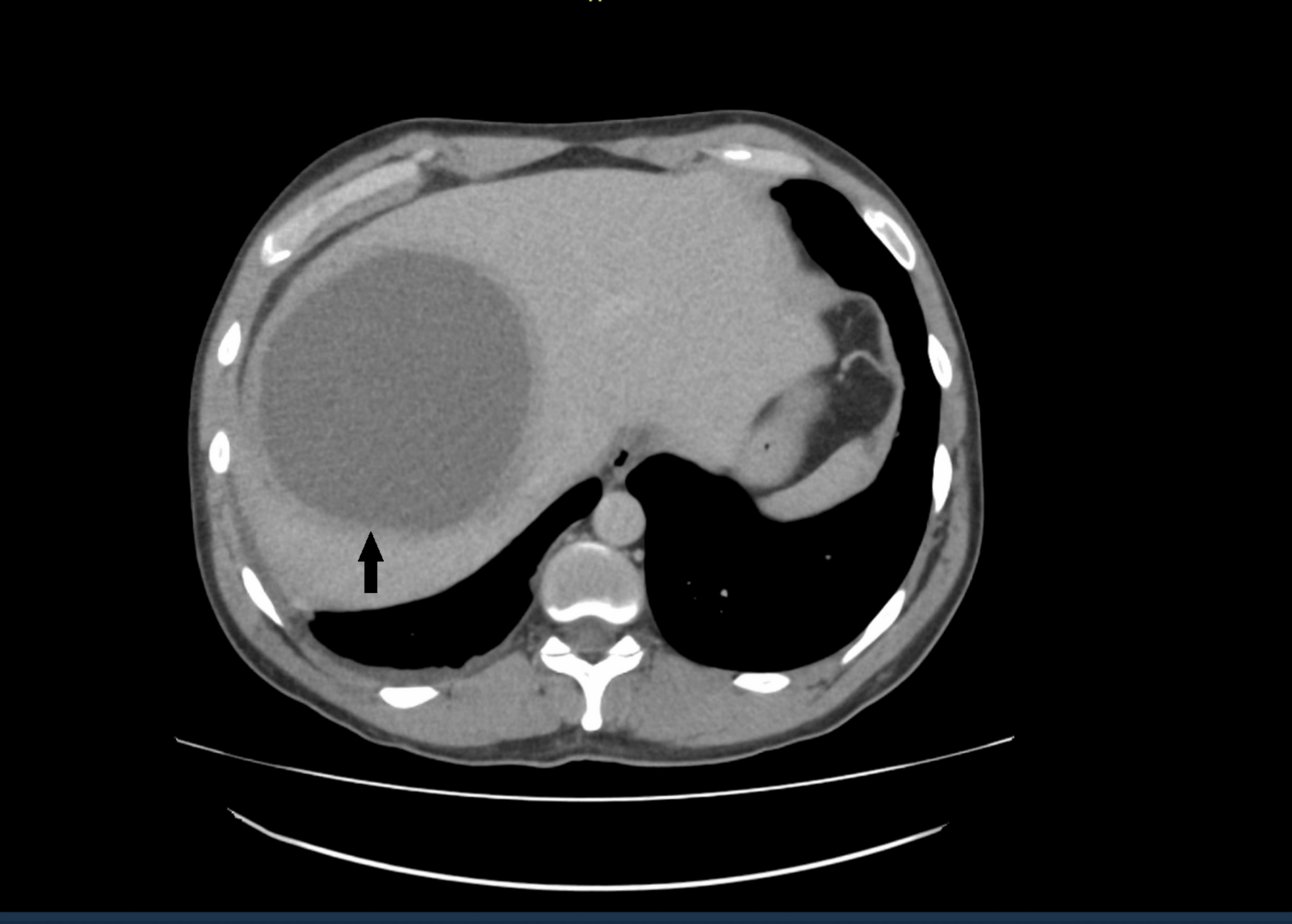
A computed tomography scan with contrast of the abdomen A cystic lesion in the right hepatic lobe with a thick-walled hepatic lesion measuring 12 cm x 11 cm can be seen exerting a local mass effect (black arrow).
